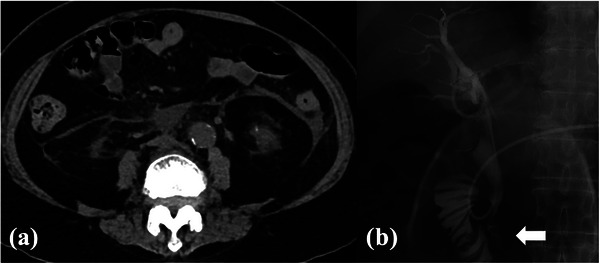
(a) Computed tomography the day after endoscopic retrograde cholangiopancreatography showed inflammatory spread to the anterior pararenal space due to pancreatitis. (b) Cholangiography from the endoscopic nasal biliary drainage tube showed a small amount of contrast medium surrounding the papilla as shown using the arrow, but no leakage into the abdominal cavity.
